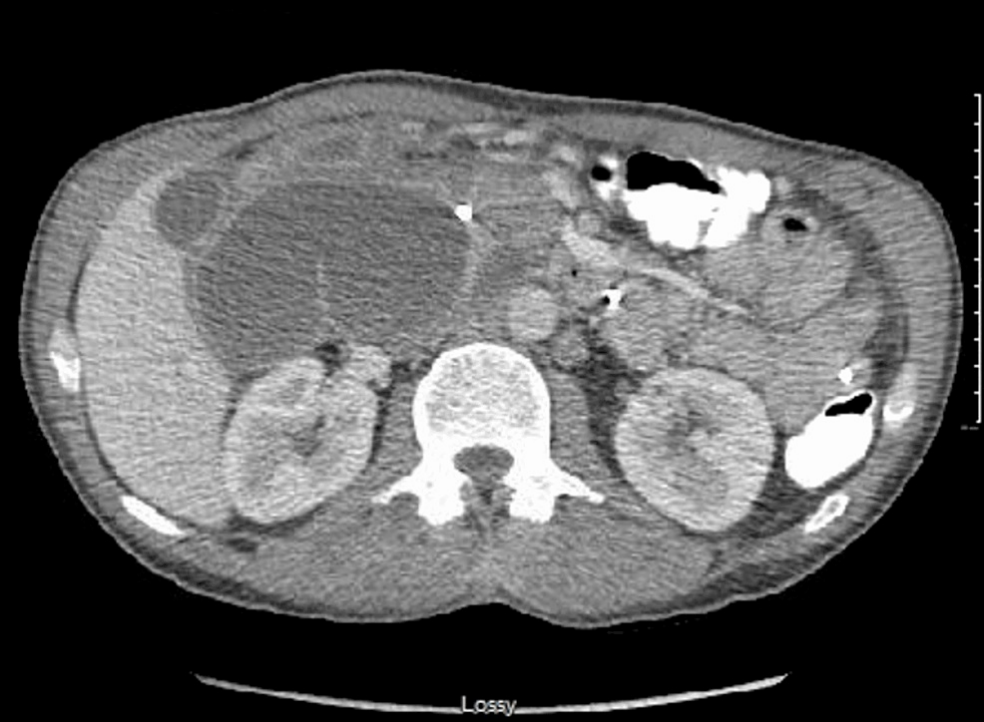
CT abdomen with contrast showed that, between the pancreatic head and gallbladder, there is a large multilocular cystic collection with an enhancing wall, which continues to increase in size, measuring at least 10.6 cm in the greatest axial dimension
